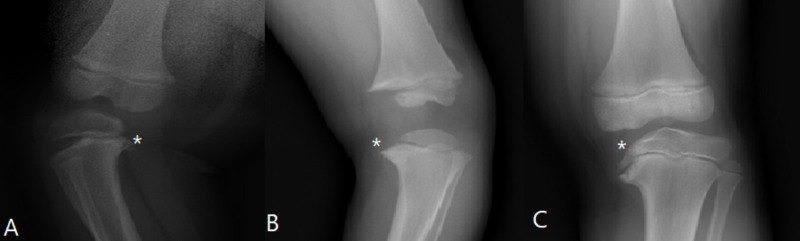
X-rays demonstrating an example of Types A, B, and C according to the LaMont et al. classification for Blount’s disease LaMont et al. [8]
